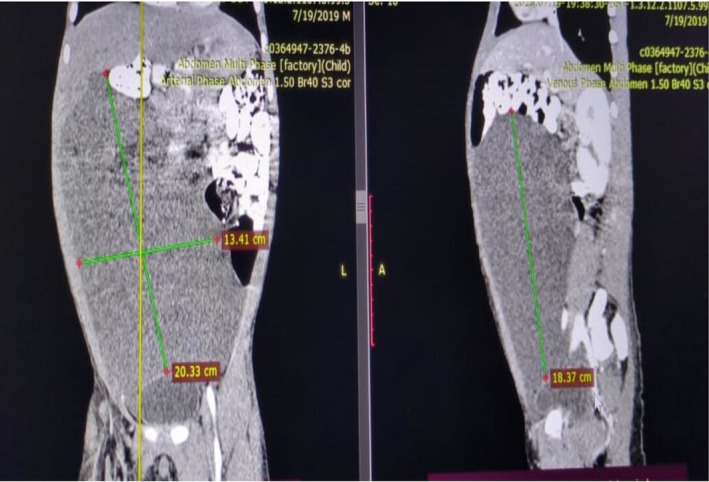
CT‐Scan revealed a huge mesenteric cyst measuring approximately 20 × 18 × 13 cm in size with fluid contents.
